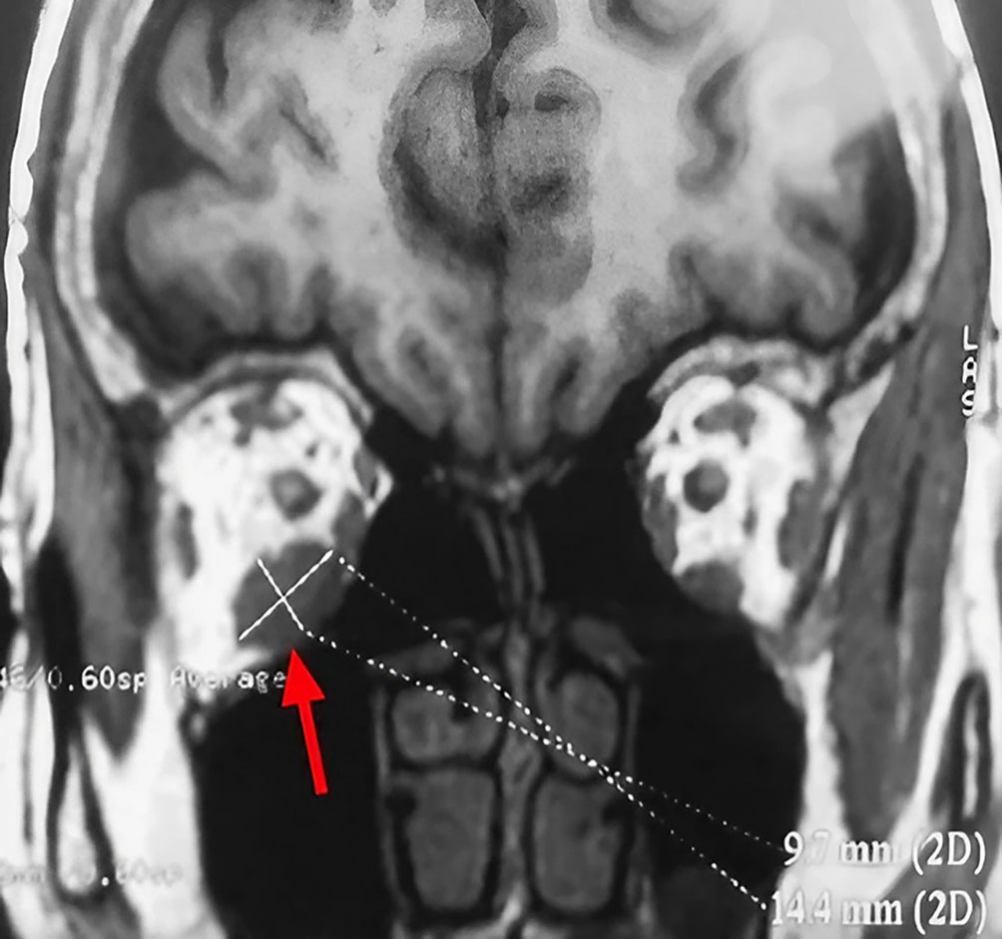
Coronal section of orbital MRI showing a mass with low signal on a T1 weighted image (red arrow).
